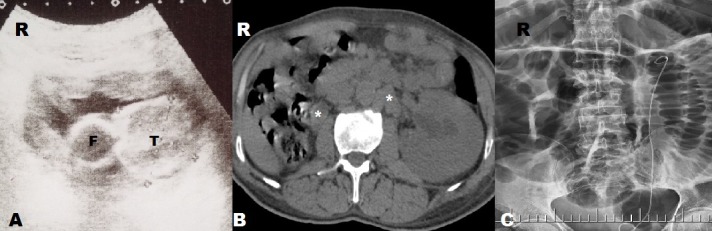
Radiological findings: A) bladder ultrasonography T: tumor; F: Foley catheter; B) non-contrast-computed tomography image, lymph node swelling (asterisks); C) JJ stent after transurethral resection
